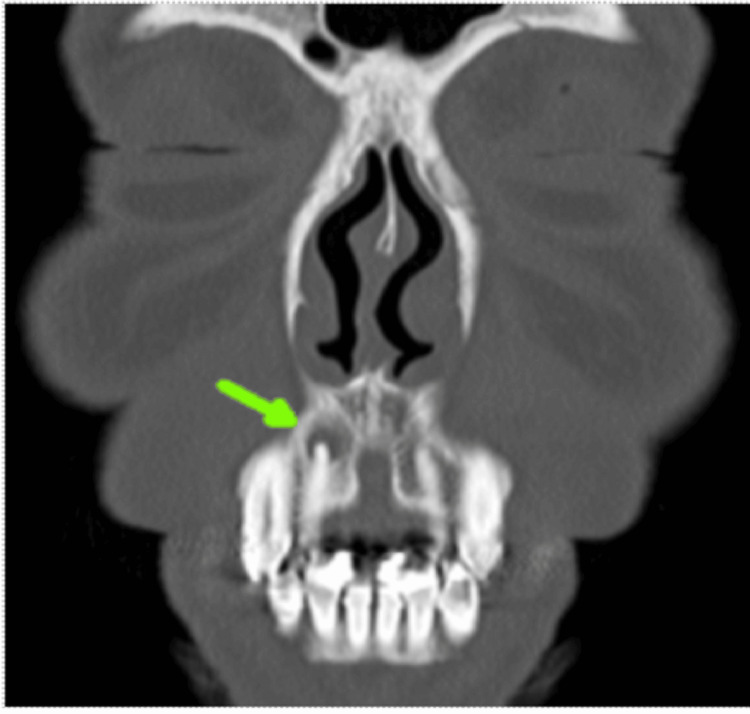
Dental computed tomography demonstrating the presumed odontogenic source of bacteremia Dental computed tomography revealed a periapical cystic lesion associated with the right maxillary lateral incisor (arrow). The crown was fractured, and the tooth was non-vital. In addition, generalized alveolar bone resorption involving both the maxilla and mandible was observed, consistent with chronic periodontitis. These findings were considered the most likely source of *Prevotella denticola *bacteremia.
